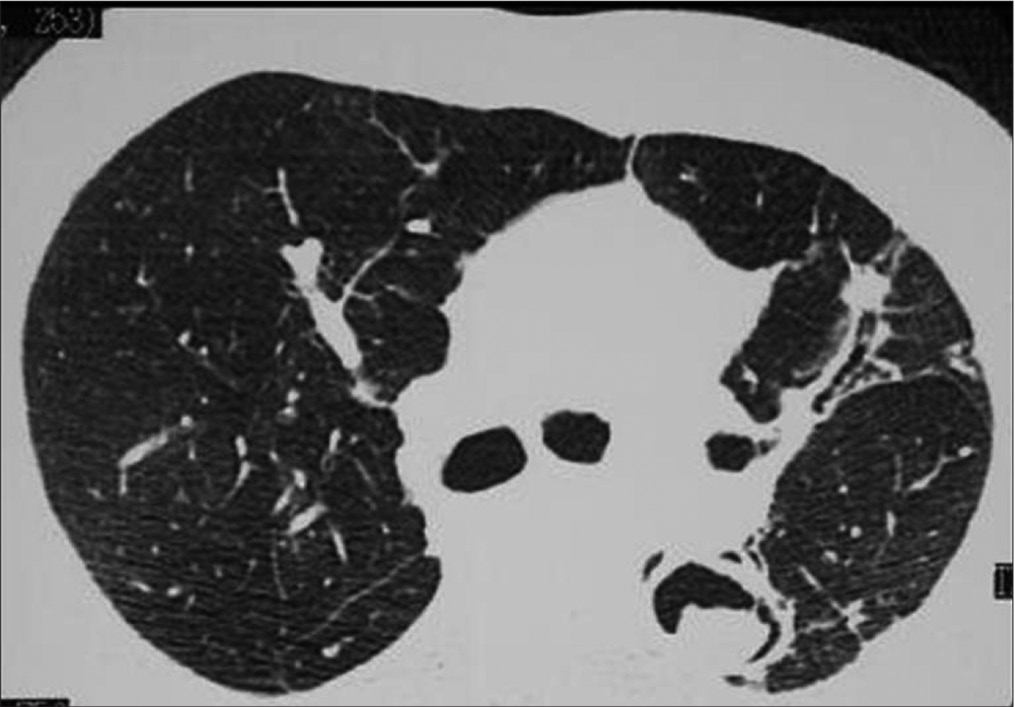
Aspergillosis. Mycetomas are the least common, but can complicate cavitary *Mycobacterium tuberculosis* or *Pneumocystis carinii* pneumonia. The computed tomography here represents a mycetoma in a tuberculous cavity. Note the traction bronchiectasis and loss of volume in the left upper zone
